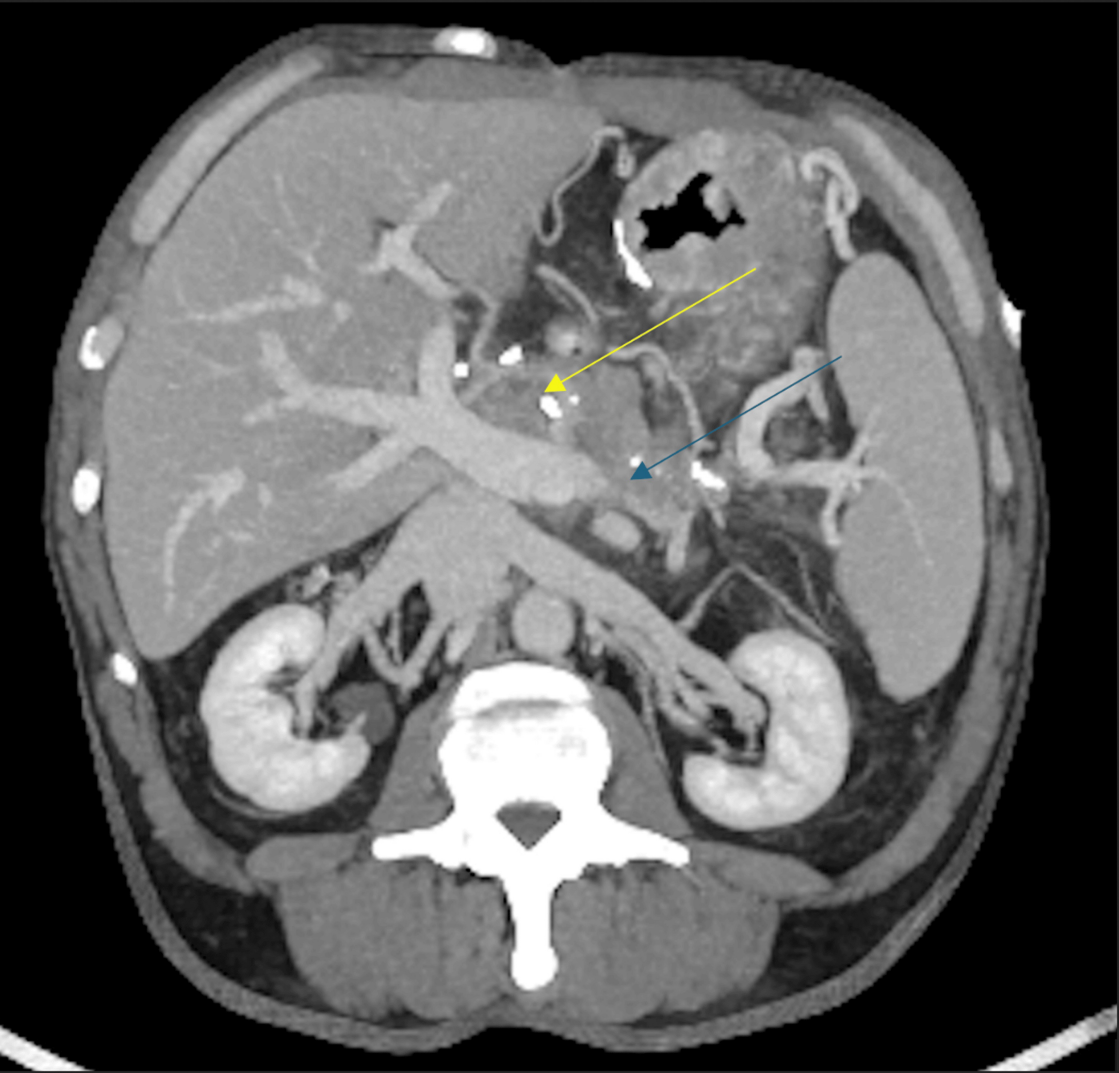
The axial, oblique view of the CT scan with IV contrast in the portal venous phase demonstrates splenic vein thrombosis and calcifications in this patient's status post Whipple procedure. The blue arrow demarcates the splenic vein thrombosis. The yellow arrow demarcates calcifications that resulted from the previous Whipple procedure.
